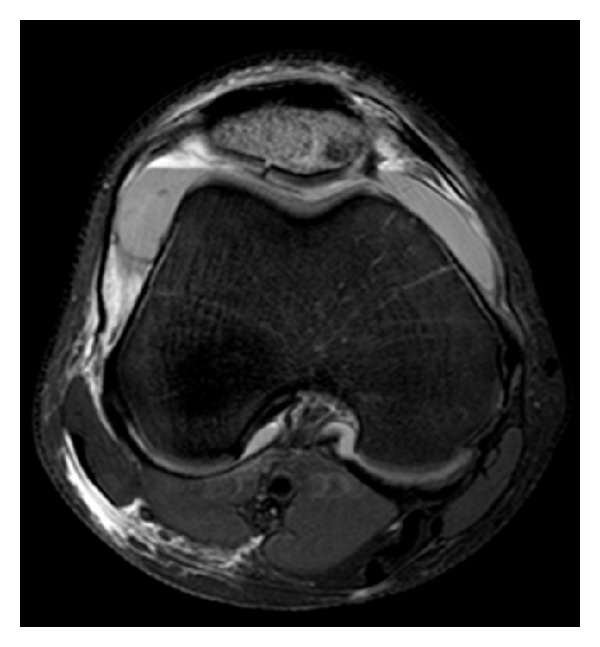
Axial T1 weighted magnetic resonance image taken one week after presentation.
